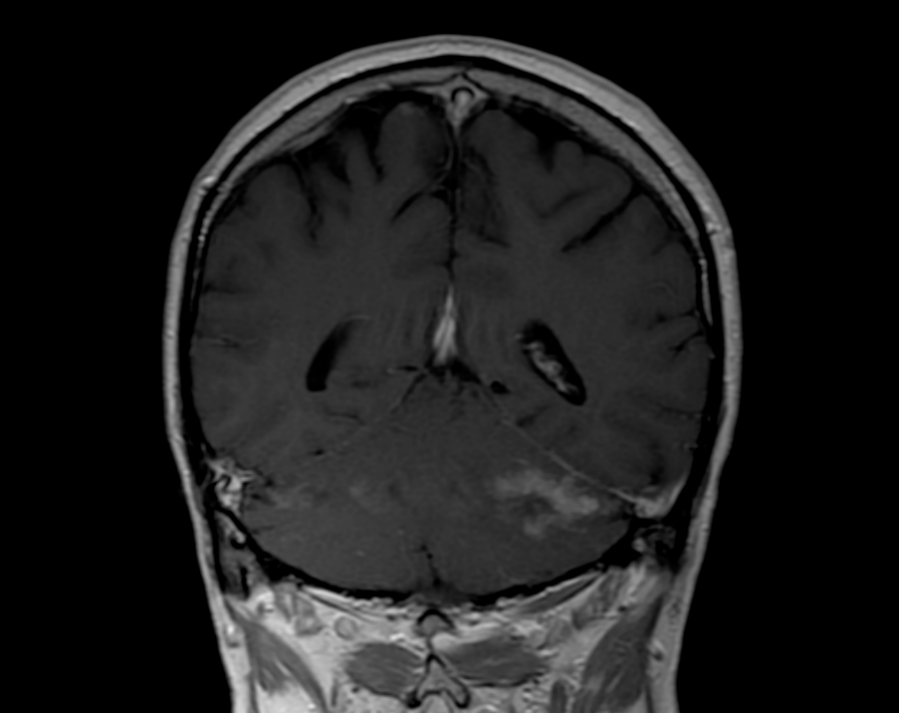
MRI brain of patient 1 before treatment showing a lesion in the cerebellum
